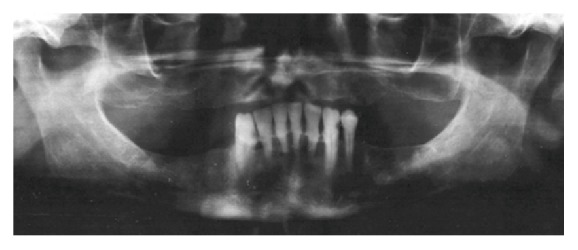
Panoramic radiograph showing horizontal bone loss in the region of mandibular anterior teeth, consistent with chronic periodontitis.
